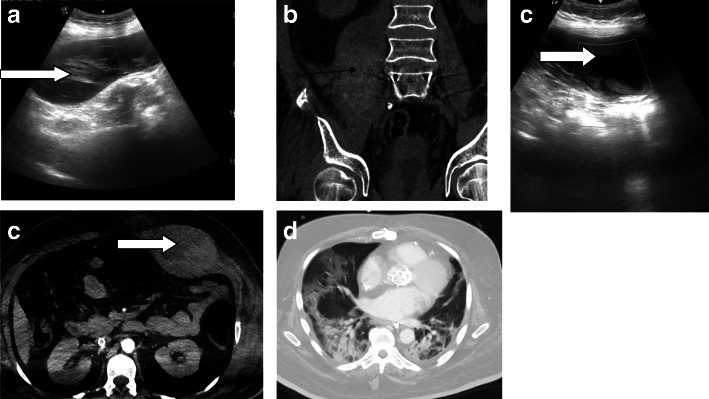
Two different COVID19 patients with spontaneous hematomas (a) sonographic assessment of the right psoas spontaneous hematoma (black arrow in a and b) in COVID-19 patient with corresponding (b) non-contrast CT scan (c) left rectus sheath hematoma (white open arrow in c and d) with (d) corresponding CT image in the arterial phase with no contrast extravasation (e) basal chest scans in the second patient showing bilateral basal patches of consolidation of COVID-19 pneumonia
